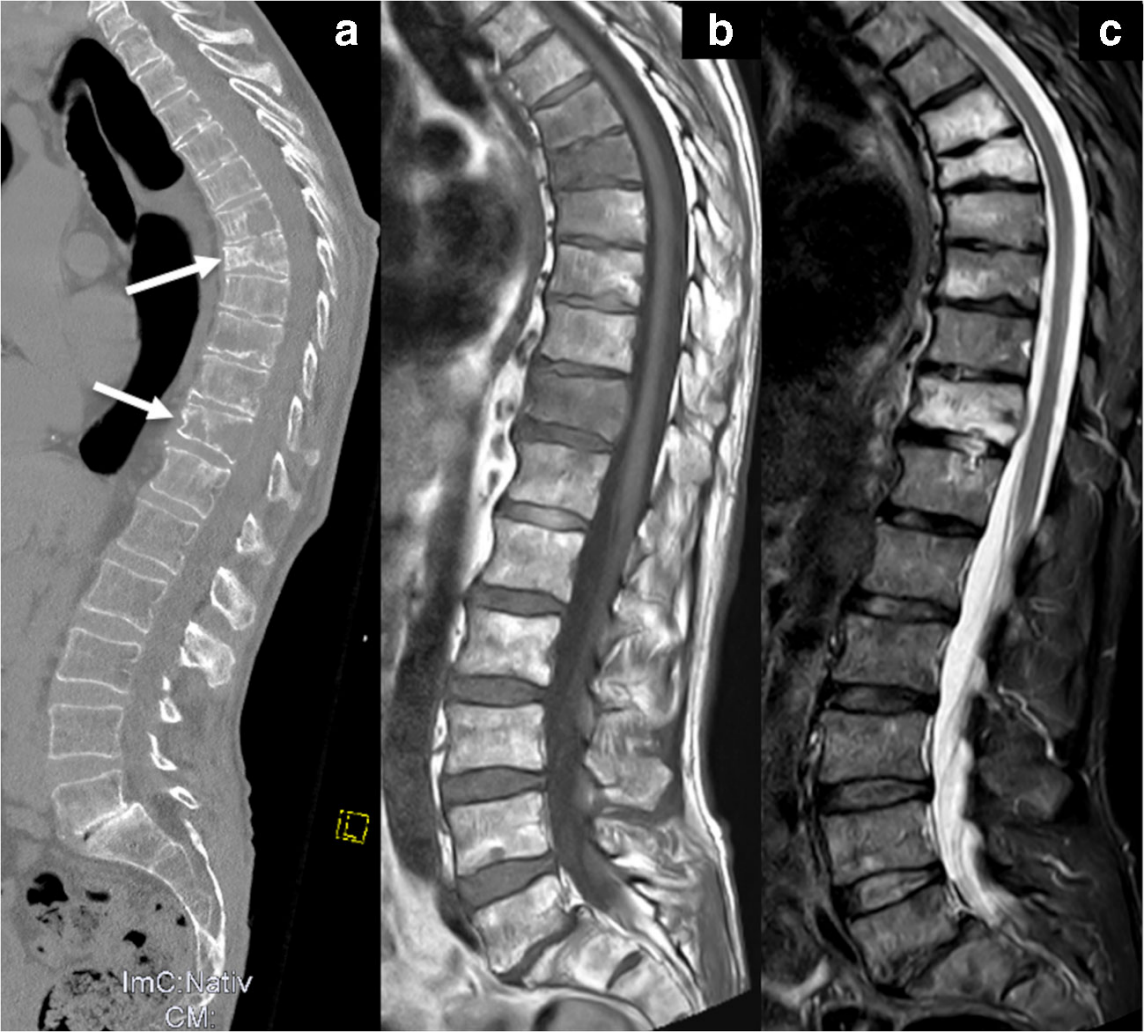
(a) Marked osteolytic lesions are detected in the 6th, 7th and 11th thoracic vertebral body and pathological fractures in the 7th and 11th vertebrae in the sagittal CT reconstruction of the spine in the bone window. Additional lesions are suspected in the 8th–10th vertebral body. MRI of the patient using a T1-weighted (b) and a STIR (c) sequence in sagittal orientation confirms the involvement of myeloma in the 6th, 7th and 11th vertebrae, while end plate-associated edema due to degenerative disease is seen in the 8th and 9th thoracic vertebrae. Thus, CT can sometimes over-diagnose myeloma involvement in cases of osteoporosis and inhomogeneous bone structure
